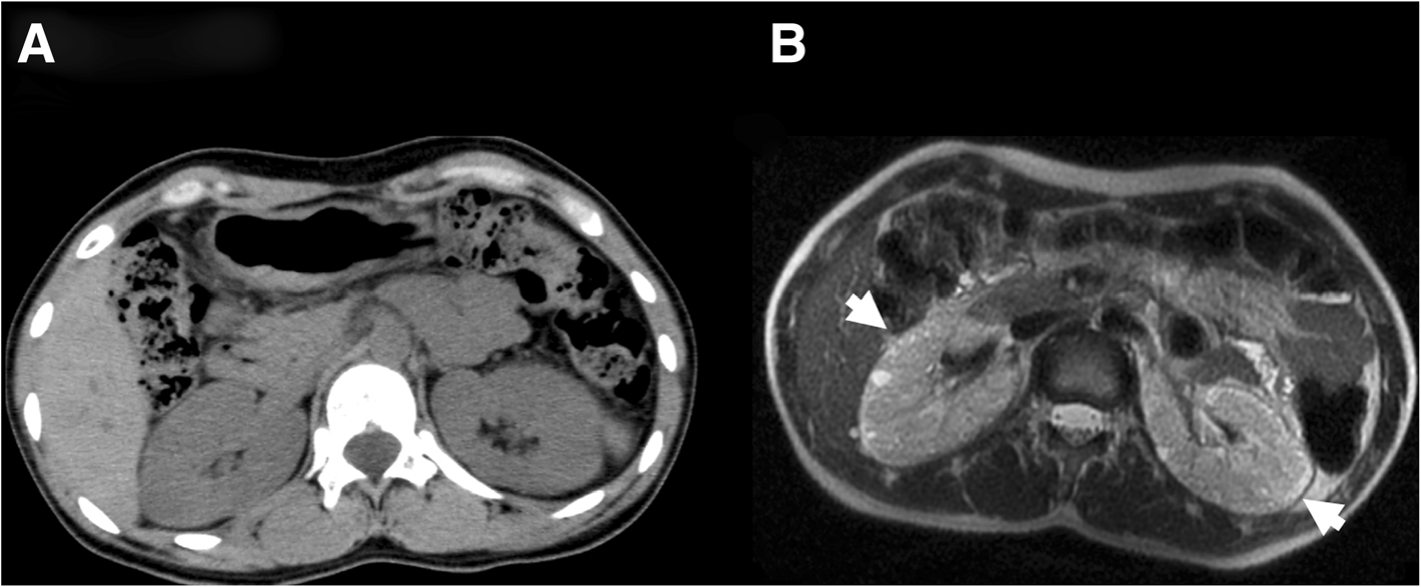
Kidney morphology. a Computed tomography scan of the kidney. The density of the renal cortex was remarkably low in the bilateral, normal sized kidneys. b Magnetic resonance imaging (MRI) of the kidneys. Numerous small high intensity spots (arrow) were distributed within the subcapsular cortex on T2-weighted MRI
